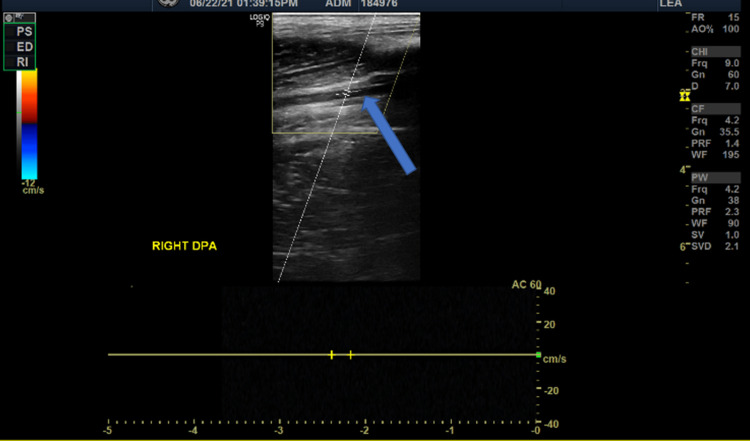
Doppler image shows absent blood flow in right dorsalis pedis artery (blue arrow)
